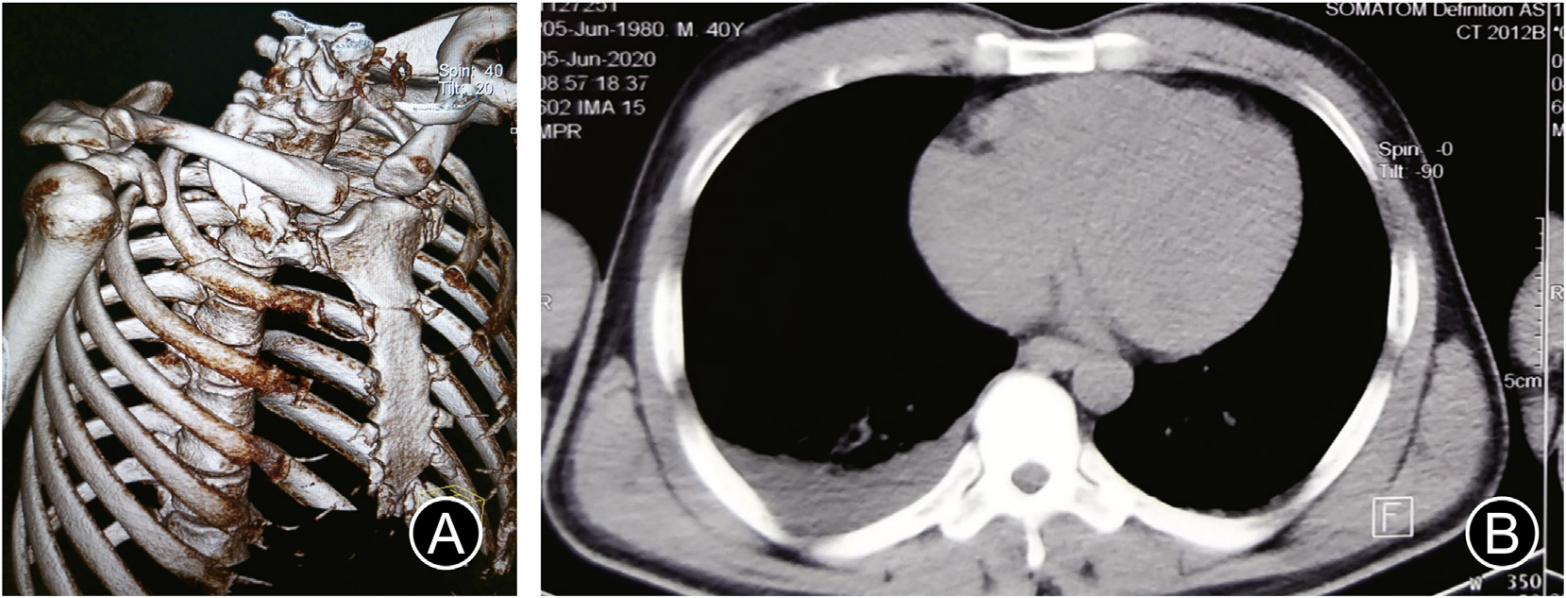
Radiological findings in elephant attack: (A) 3D reconstruction of CT chest showing 1st and 2nd rib-fracture; (B) Cross-sectional image of CT thorax showing haemothorax.
